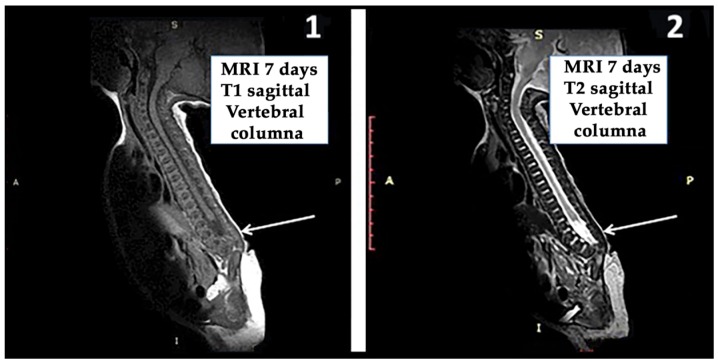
Magnetic resonance imaging (MRI) of the vertebral column MRI performed at 7-days of age. (1) The white arrow shows that the vertebral column development had been interrupted at the L2–L3 level and that the spinal cord was tethered; (2) A small lipoma can be seen at the end of the conus medullaris (arrow); there was sacral agenesis too. A: anterior; P: posterior; S: superior; I: Inferior. T1, the time when 63% of the longitudinal magnetization has recovered; T2, the time when 63% of the transverse magnetization has decayed.
